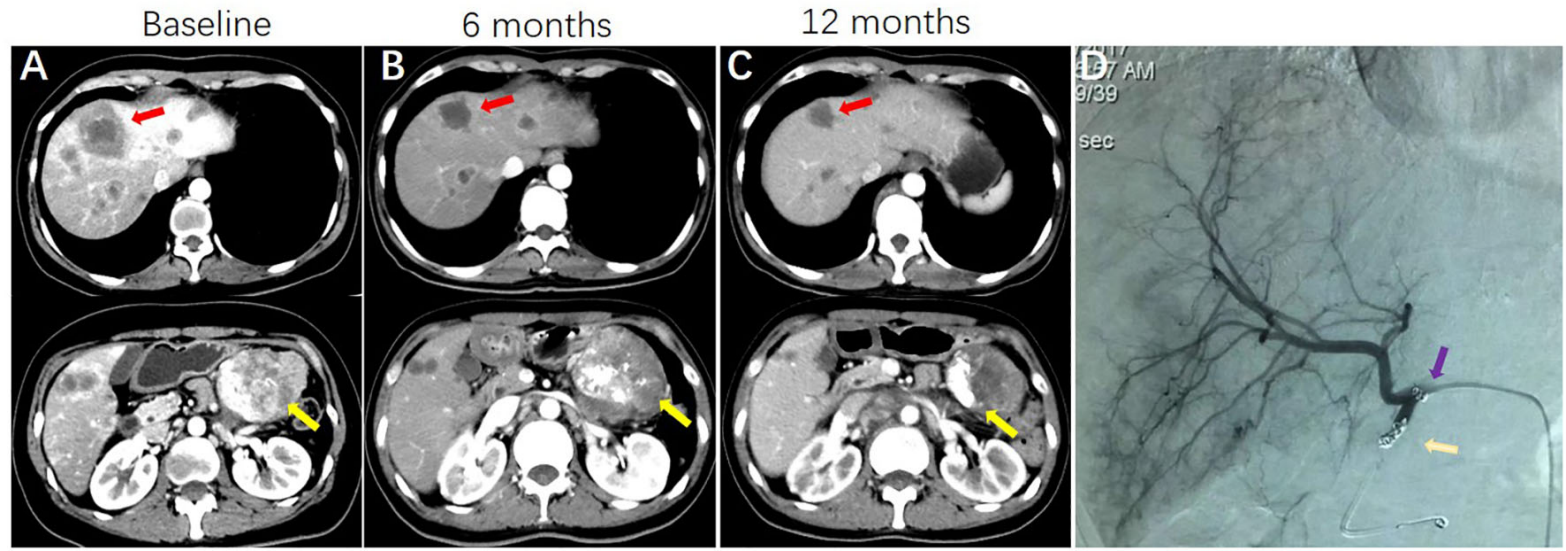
A patient with pancreatic cancer and synchronous liver metastases, who was treated with systemic GC HAI FUDR. (A) CT images showing the presence of pancreatic cancer (yellow arrows indicate) and synchronous liver metastases (red arrows indicate) at baseline. (B) CT images show primary cancer and liver metastases are shrinking at 6 months. (C) images show sustained response of liver metastases and primary cancer. (D) Hepatic arteriography shows HAI pump functionality at 12 months (purple arrow indicates side hole of the catheter; gold arrow indicates the tip of the catheter, which was located in the occluded gastroduodenal artery).
